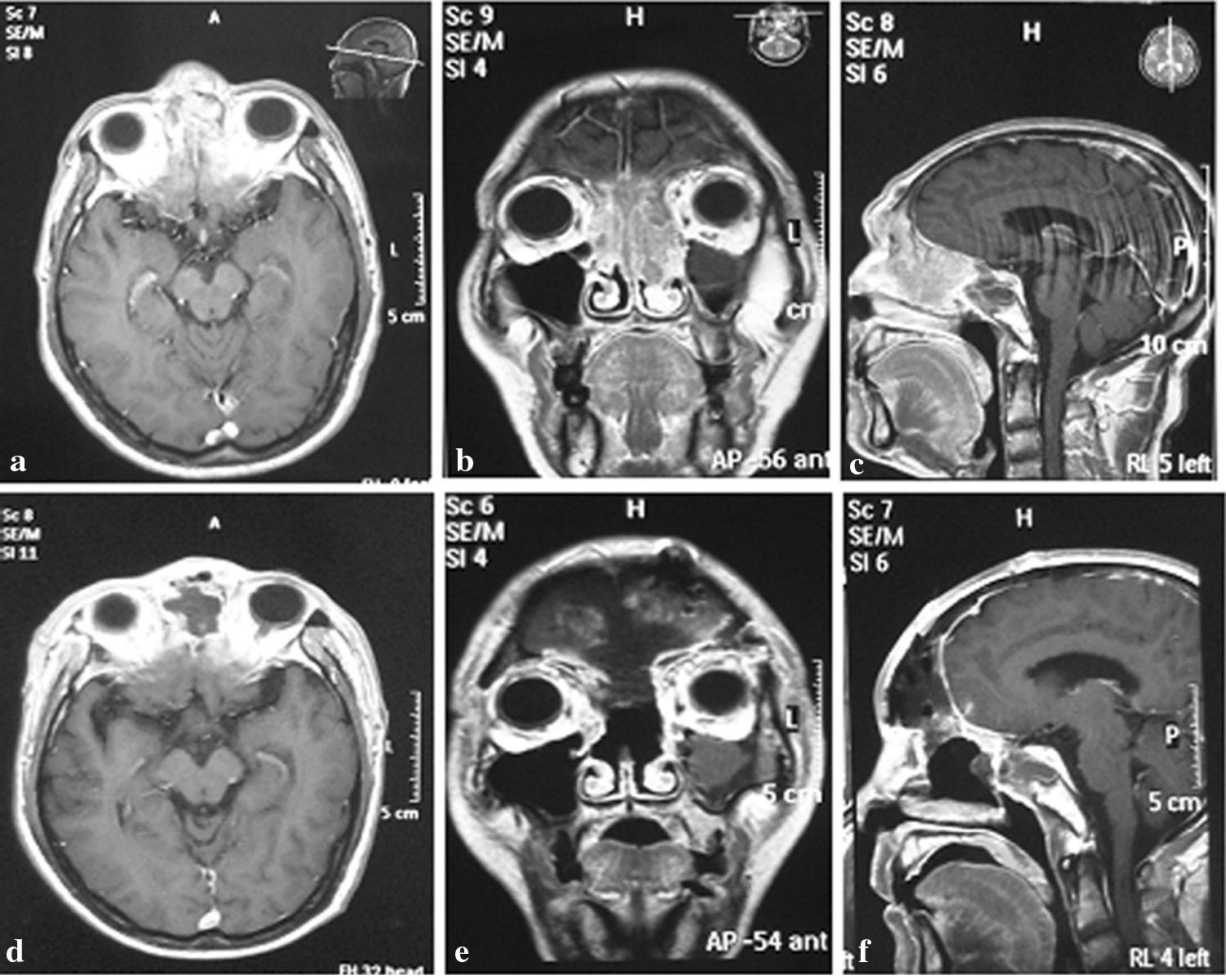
Central lesion. A 54-year-old male presented with a history of headache and nasal congestion. Biopsy revealed an inverted papilloma. a–c Preoperative magnetic resonance imaging (MRI) showed the tumor invading the frontal–nasal–orbital region, and the frontal bone at the nasion was deformed. d–f MRI images 3 months after surgery. The tumor and the deformed bone were removed, and the reconstruction of the bone defect was accomplished with a titanium meshType III or extensive (*n* = 8). The tumor was massive and located at the anterior-medial skull base, growing extensively in a nasopharyngeal, intraorbital, and intracranial direction. The scope of invasion was a combination of the two previously described (Fig. 3a–c).
